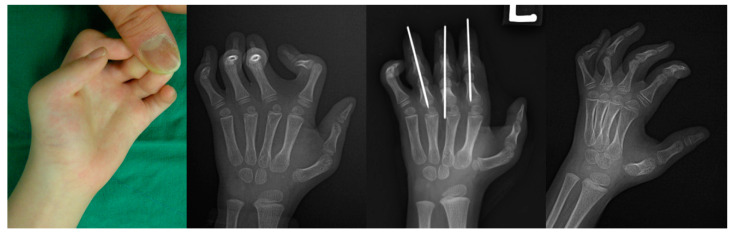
The clinical image and radiographs of an arthrogryposis patient with syndromic camptodactyly show the flexion contractures from second to fifth finger with the left hand’s thumb-in palm deformity. Despite the restricted wrist motion with the lack of wrist crease, the patient did not present the flexion contracture of the wrist but complained of discomfort when grasping objects such as cups. After the correction of camptodactyly via one-stage extension shortening osteotomies, the hand function improved with the proper bony union.
